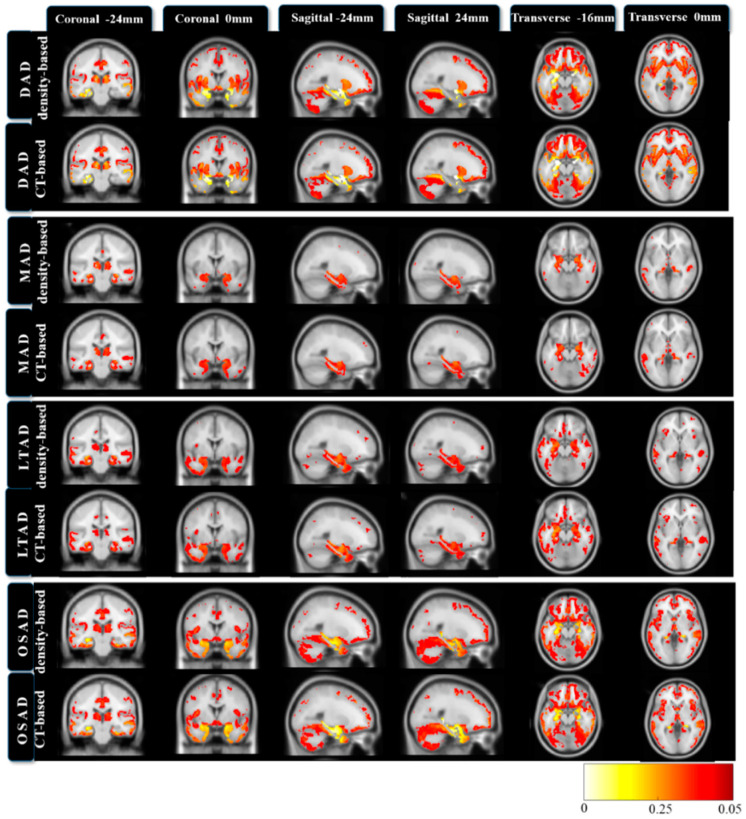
Statistical parametric maps of subtypes were identified by CT-based and density-based compared with the CNs. The results were thresholded at FDR corrected *p* < 0.05. The statistical parametric maps included all the subjects in each subtype. Abbreviations: DAD: diffuse atrophy AD subtype, MAD: minimal atrophy AD subtype, LTAD: left temporal dominant atrophy AD subtype, OSAD: occipital sparing AD subtype, CT: cortical thickness.
